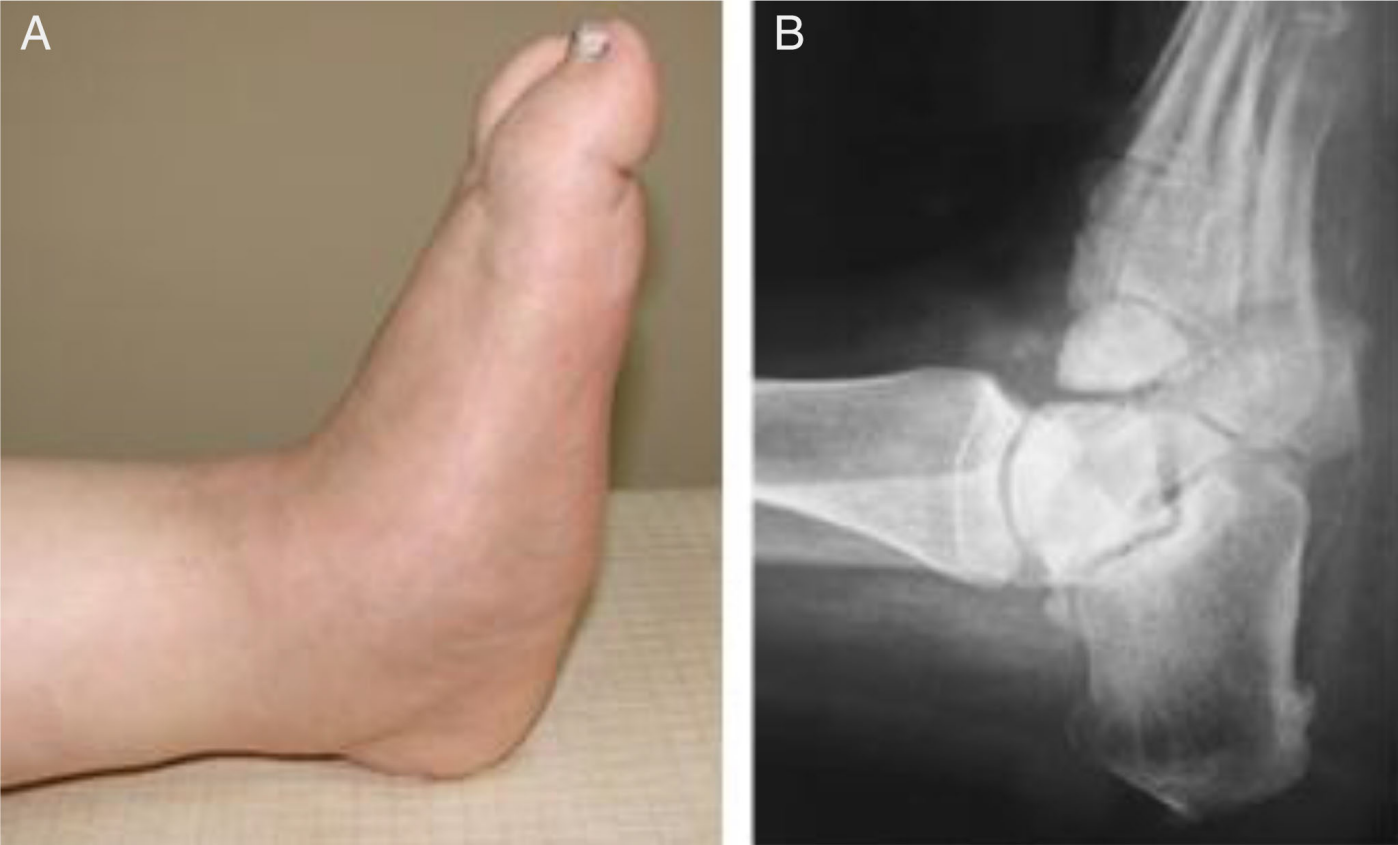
Rocker-bottom Charcot foot (a) Clinical appearance (b) Lateral radiograph.
